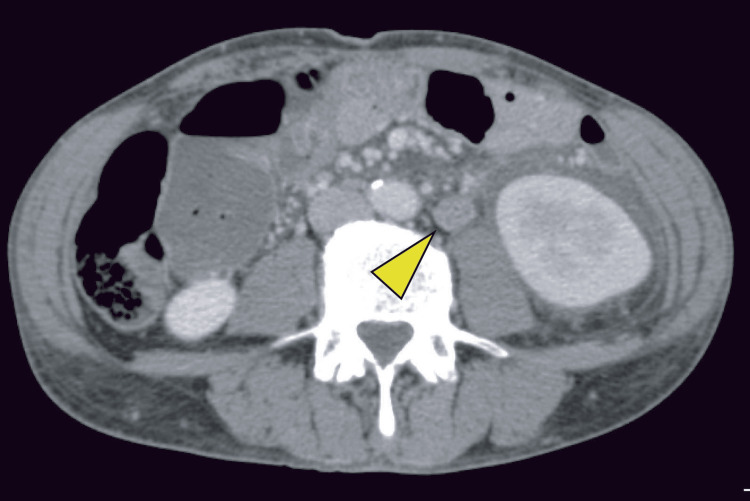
Contrast-enhanced abdominal CT on hospital day two Yellow arrows indicate newly developed retroperitoneal metastasis adjacent to the left ureter.
